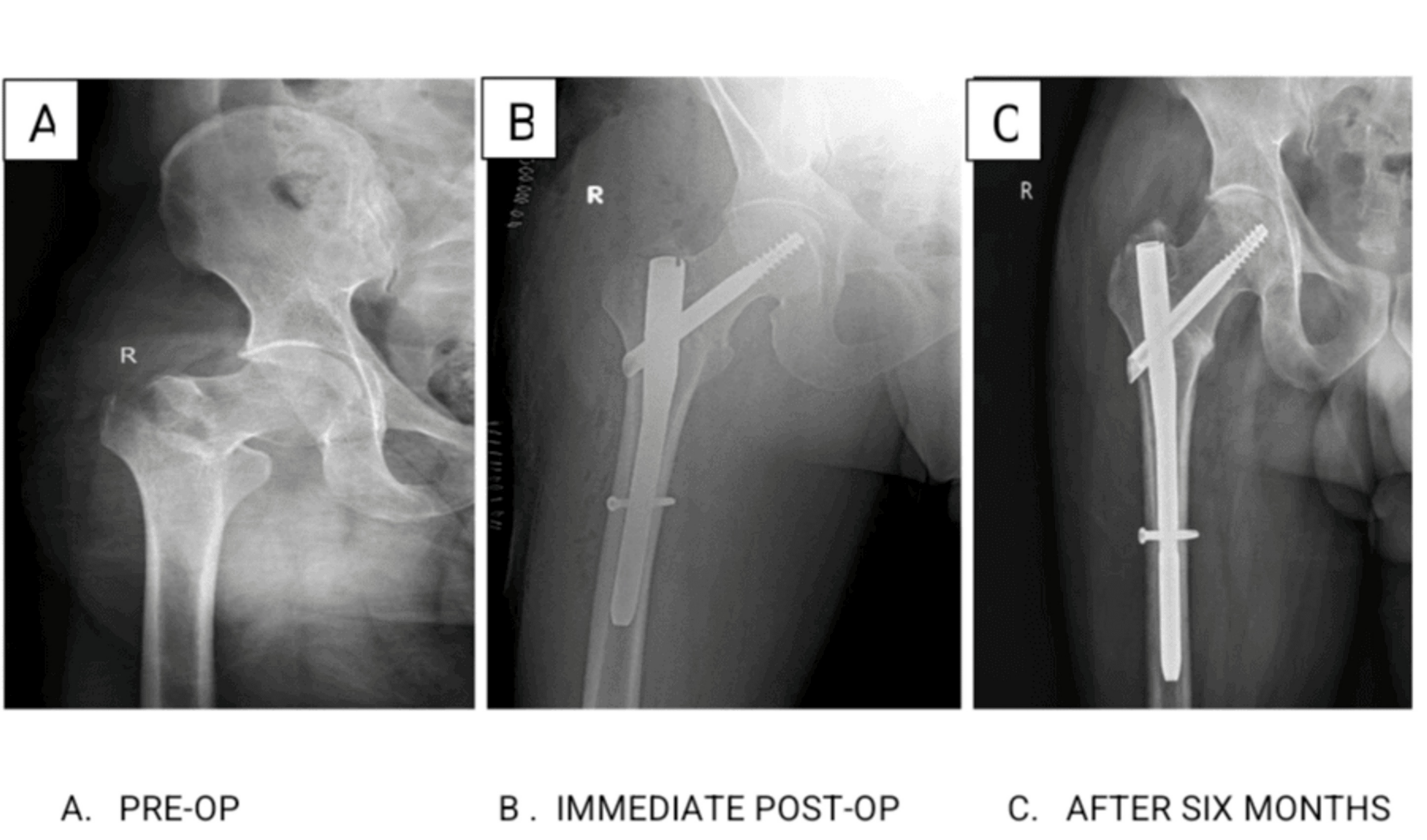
Post-operative X-ray of the patient operated using the trochanteric femoral nail.
